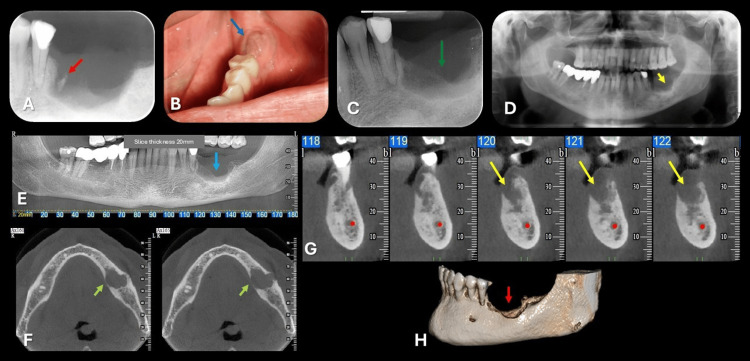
Clinical and radiographic findings of the lesion. (A) Periapical radiograph showing a retained root fragment (red arrow) at the extraction site, suggestive of local irritation and possible infection.
